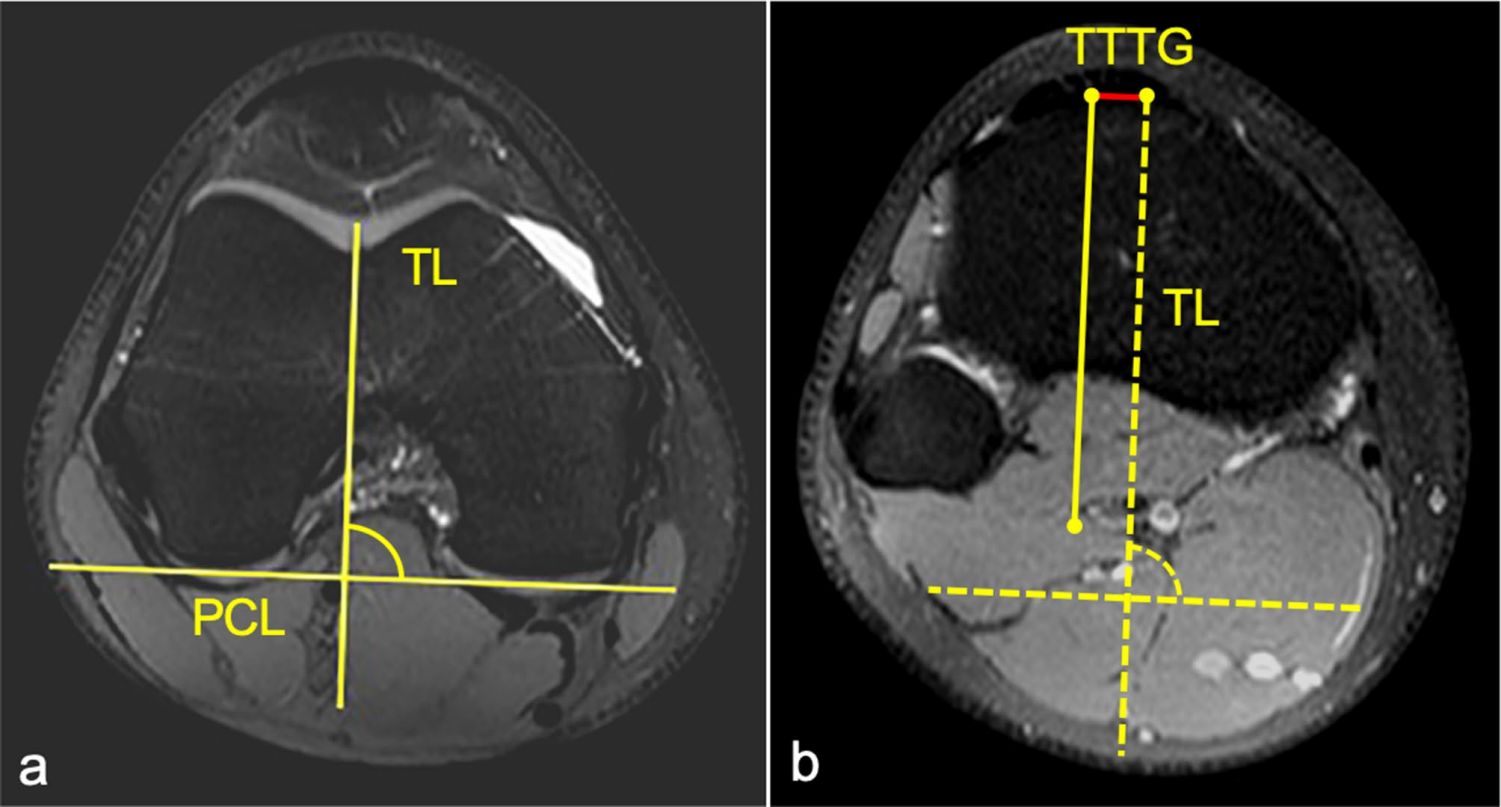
Determination of the TT–TG distance using fat suppressed PD-weighted images in transversal orientation. First, the most proximal MR image slice with full cartilage coverage of the trochlear groove is chosen to assess the trochlear groove, and the posterior condylar line is drawn tangential to the chondral border of the posterior condyles of the femur. Further, the TL is drawn perpendicular to posterior condylar line (a). Secondly, TL will be transferred to most cephalad image in which the patellar tendon is fully in contact with the tibial tubercle. The center of the tibial tubercle is marked at the midpoint of the patellar tendon on this image. The final TT–TG distance will be taken as the perpendicular distance from the center of the tibial tubercle to the transferred TL (b).
